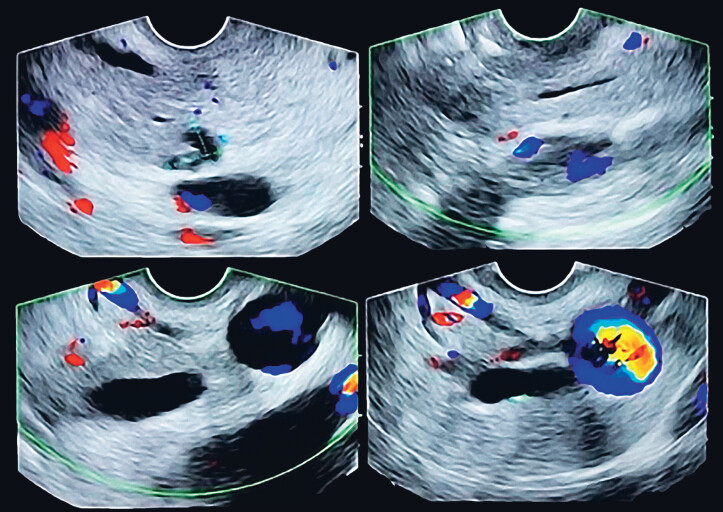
Pancreatic head cyst measuring 6×9 mm, without communication with the main or secondary pancreatic duct. 7 mm choledochus with micro calculus of 2 mm inside. Papilla are endoscopically and endosonographically normal.
